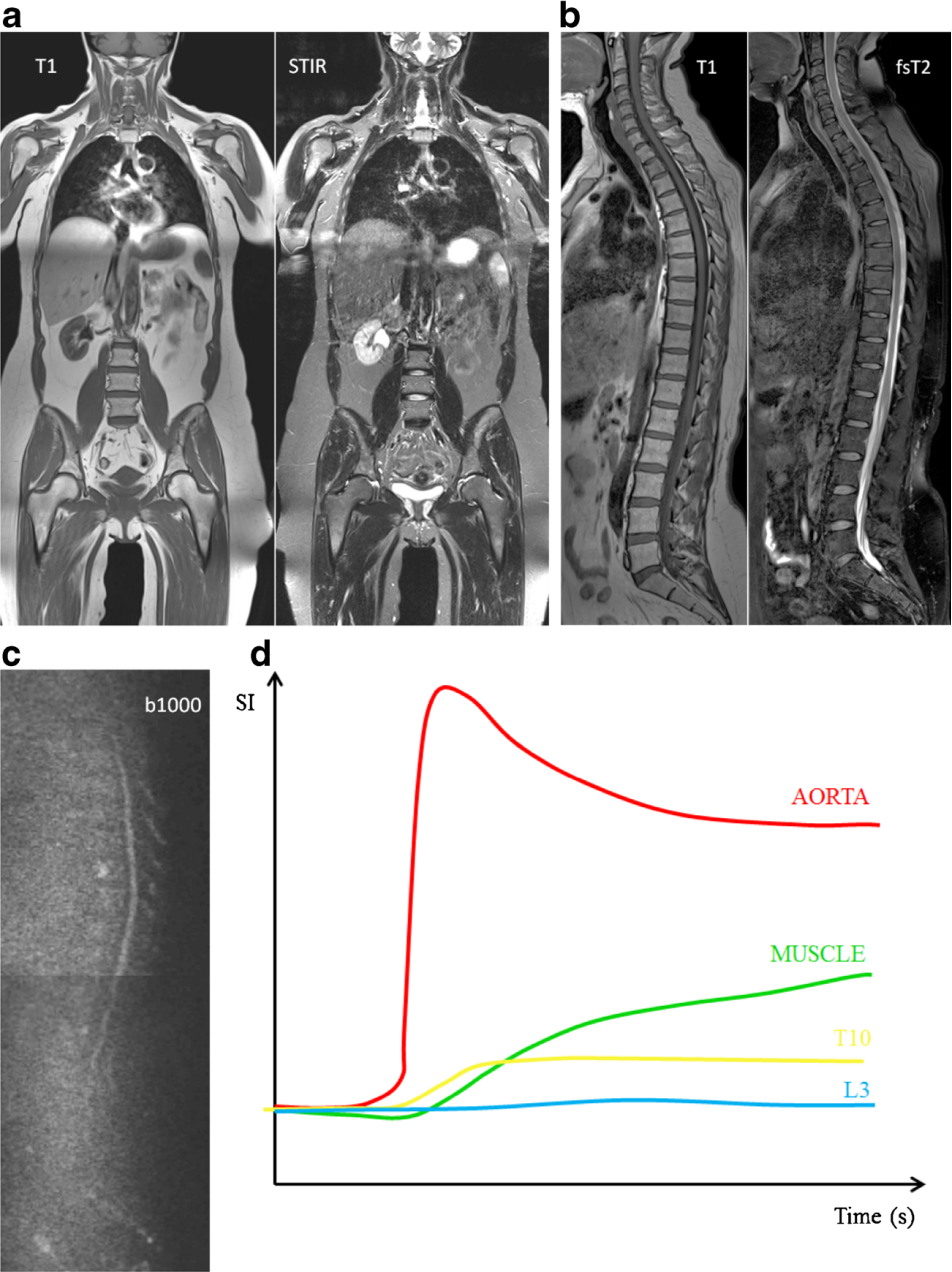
These images present the same patient as in Fig. 8, with response to therapy, 100 days after autologous stem cell transplantation, with 5 % plasma cells on bone marrow on biopsy. a T1-weighted and T2-weighted STIR coronal images of the body and b T1-weighted and fat-suppressed T2-weighted images of the thoracolumbar spine, demonstrating a clear increase in signal intensity on T1-weighted and decrease on T2-weighted images, due to reappearance of normal bone marrow. c There is a residual high signal intensity on the b1000 images, due to residual oedema and necrosis after therapy despite the return of fat cells, accompanied by d a decrease in perfusion due to destruction of the neovasculature with the reappearance of a type 1 (L3 - blue) and type 2 (T10 - yellow) curve
